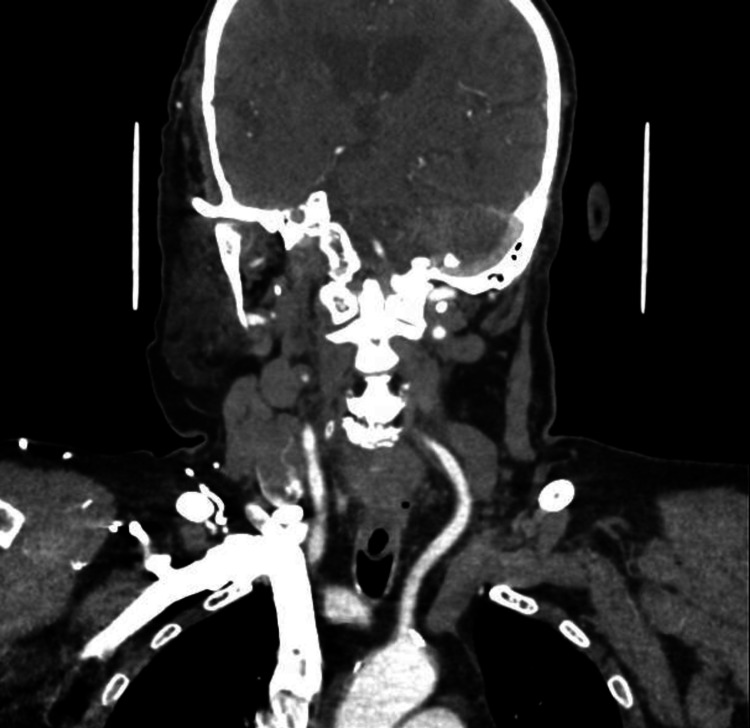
CT angiogram (sagittal view) showing the right vertebral artery with significant stenosis at its origin.
